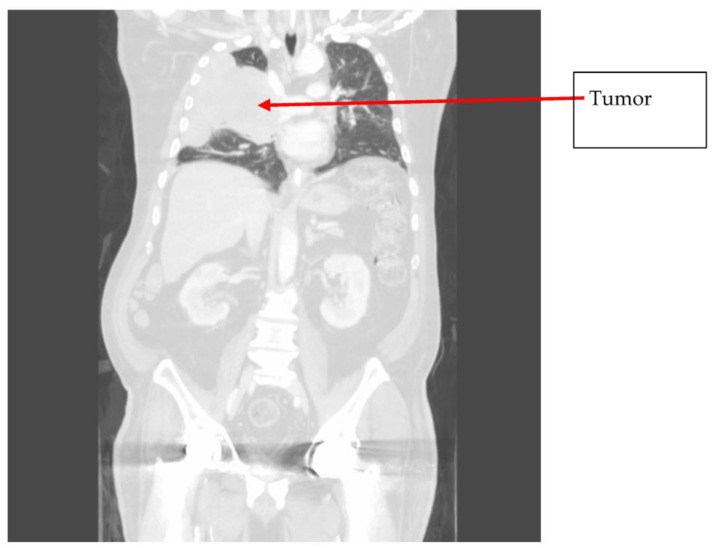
Chest CT showing extensive tumor of the right upper lobe.
